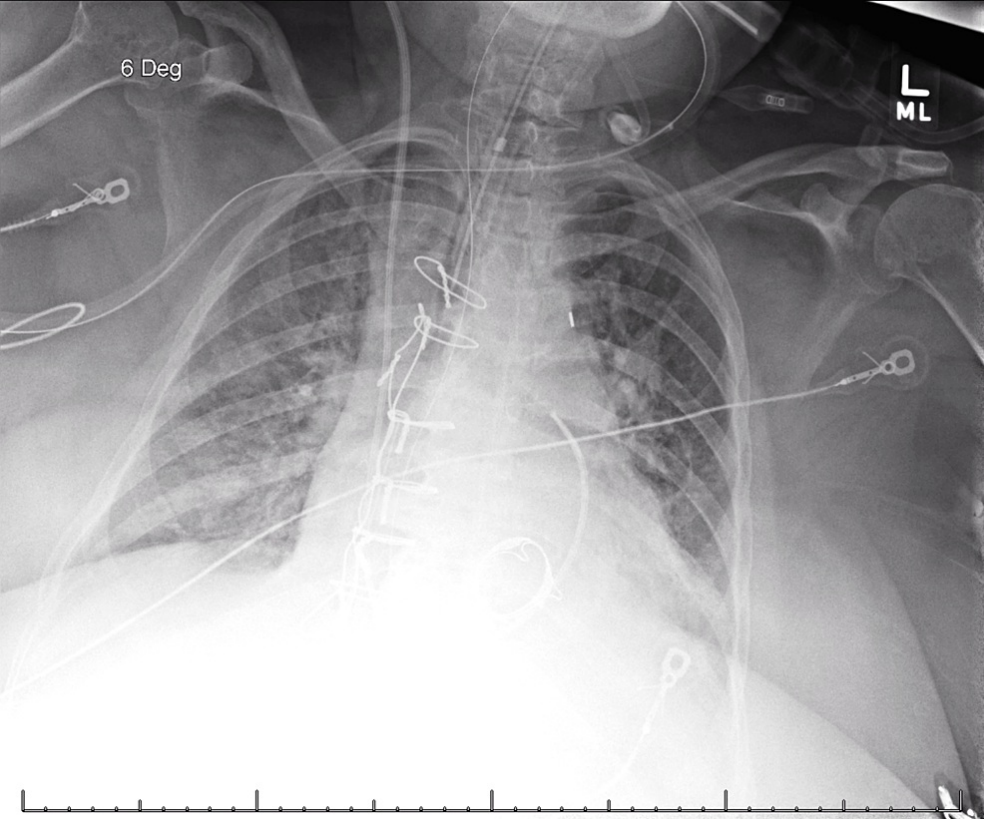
Post-operative chest radiograph (approximately 36 hours after presentation) showing significant improvement in right-sided infiltrates after mitral valve replacement.
